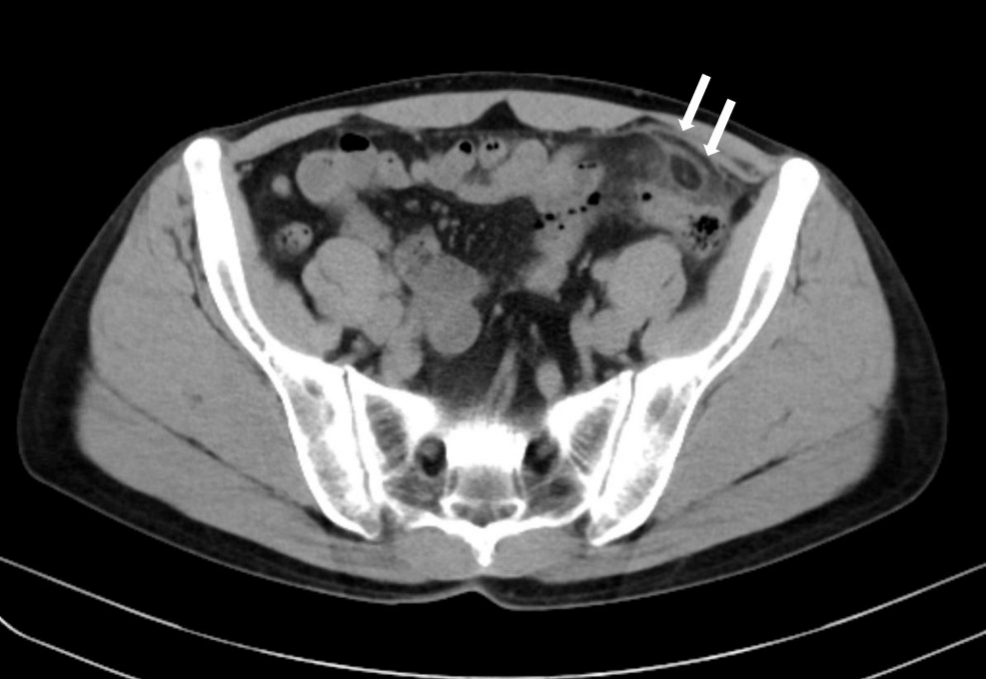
Computed tomography revealed the presence of a fat-density ovoid structure with a high-density rim adjacent to the sigmoid colon and surrounding inflammatory fat, suggesting epiploic appendagitis.
